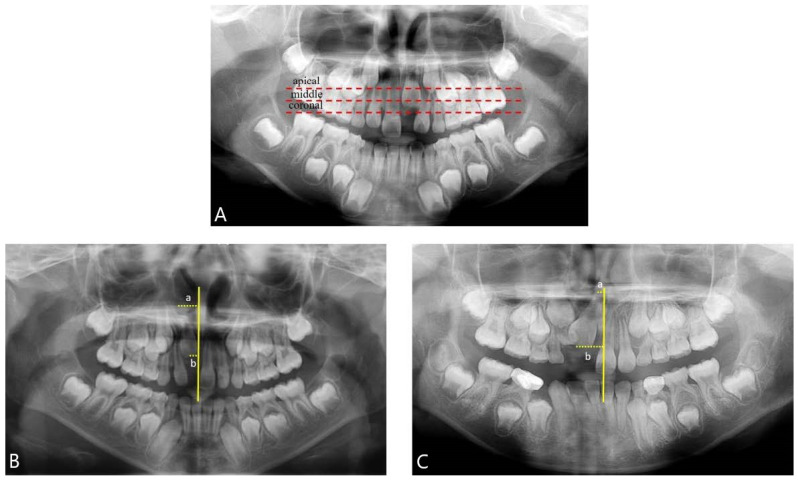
Panoramic radiographs analysis. (A) Criteria for evaluating impaction height; coronal, middle, and apical were classified with reference to the contralateral erupted central incisor. (B,C) Criteria for evaluating impaction angle (mesial, distal); using the vertical midline passing through the ANS (anterior nasal spine) as a reference, the distance from this line to the midpoint of the incisal edge (b) was subtracted from the distance from this line to the midpoint of the root apex (a) of the impacted central incisor. A positive value indicated a mesial impaction, while a negative value indicated a distal impaction.
